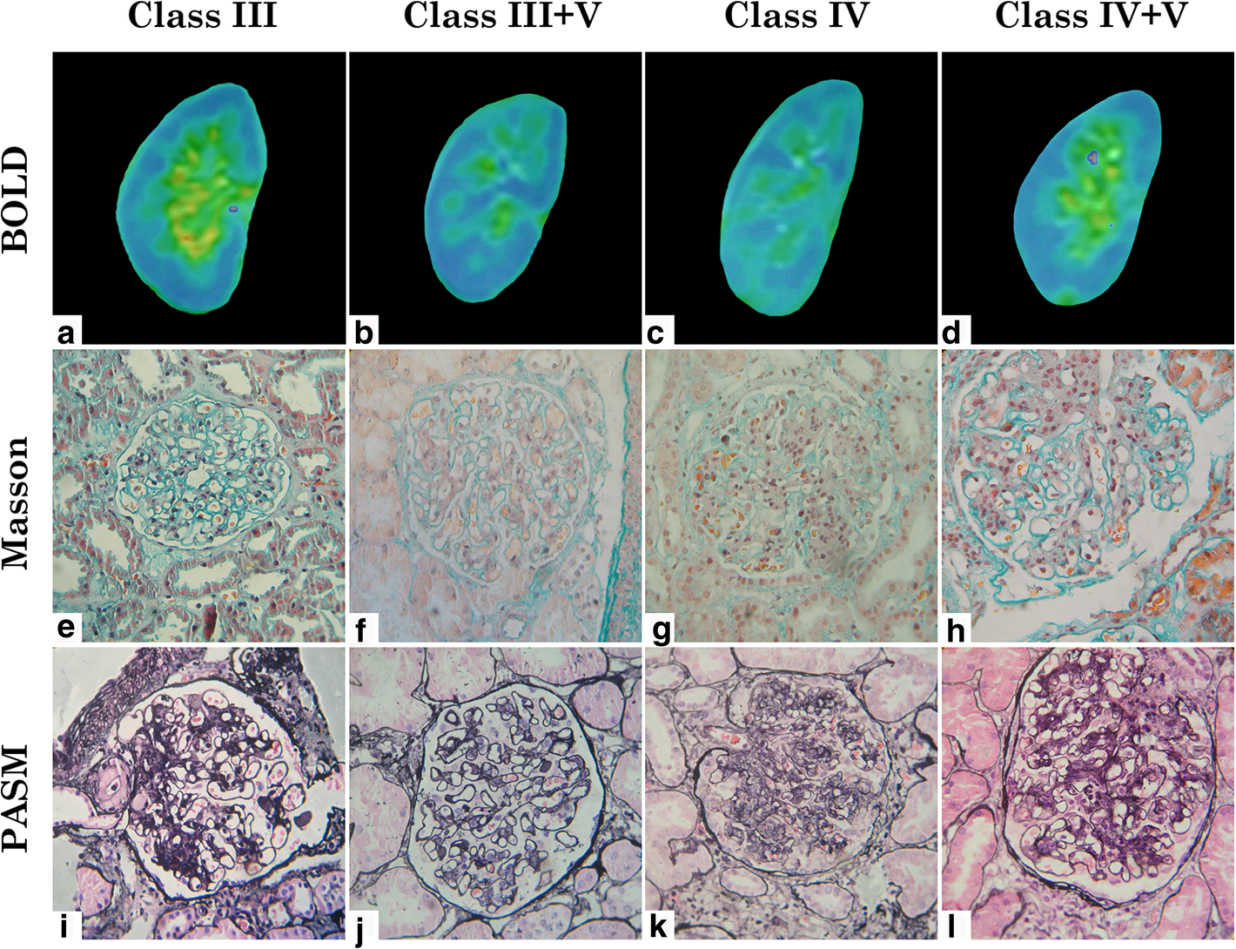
BOLD-MRI and renal pathological pictures of kidneys. Representative magnetic resonance images of a III type LN (a,e,i), III + V type (b,f,j), IV type (c,g,k) and IV + V type (d,h,l) LN patients. The BOLD-MRI pictures were expressed as pseudo-color maps. For example, blue represents the areas of lowest R2* values and oxyhemoglobin levels, whereas green, yellow, and red, in that order, represent increasing R2* values and higher oxyhemoglobin levels on the colored R2* map
